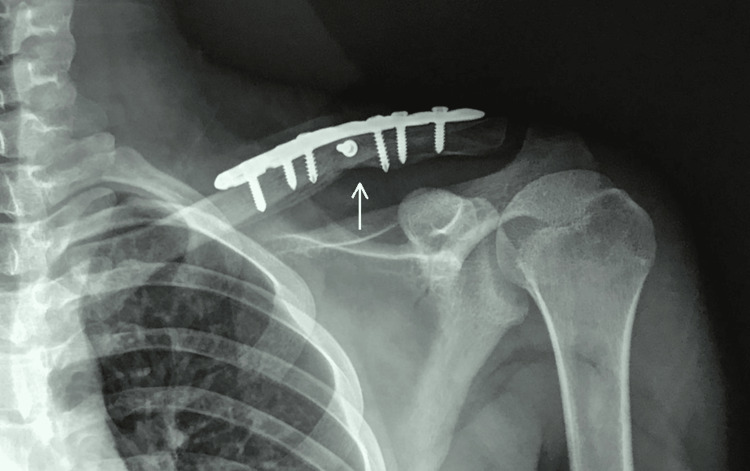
Final follow-up x-ray of patient 17. X-ray 12 weeks post-surgery demonstrating bony union (arrow).
